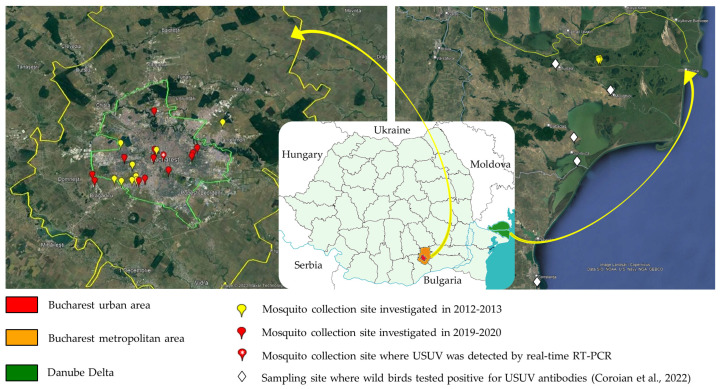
Mosquito sampling sites, South-Eastern Romania, 2012–2013 and 2019–2020. Sampling sites where wild birds tested positive for USUV antibodies were reported by Coroian et al. [16].
